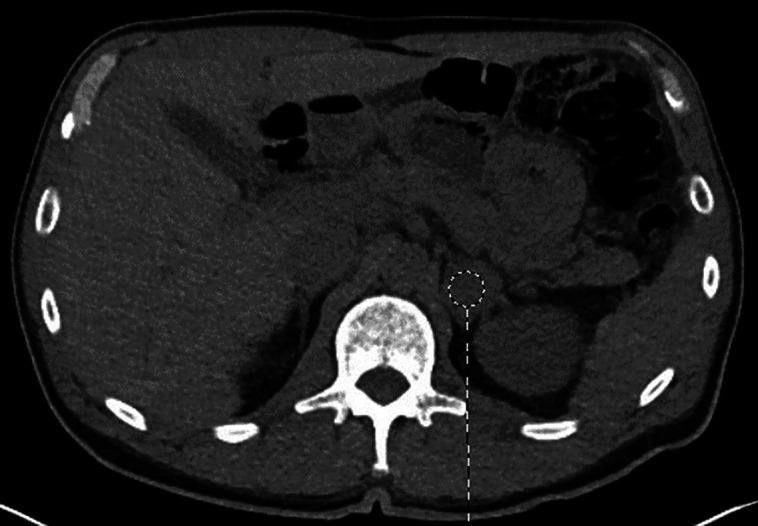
CT image of left adrenal mass (pre-contrast) of patient with aldosterone/cortisol dual-secreting ACC.
